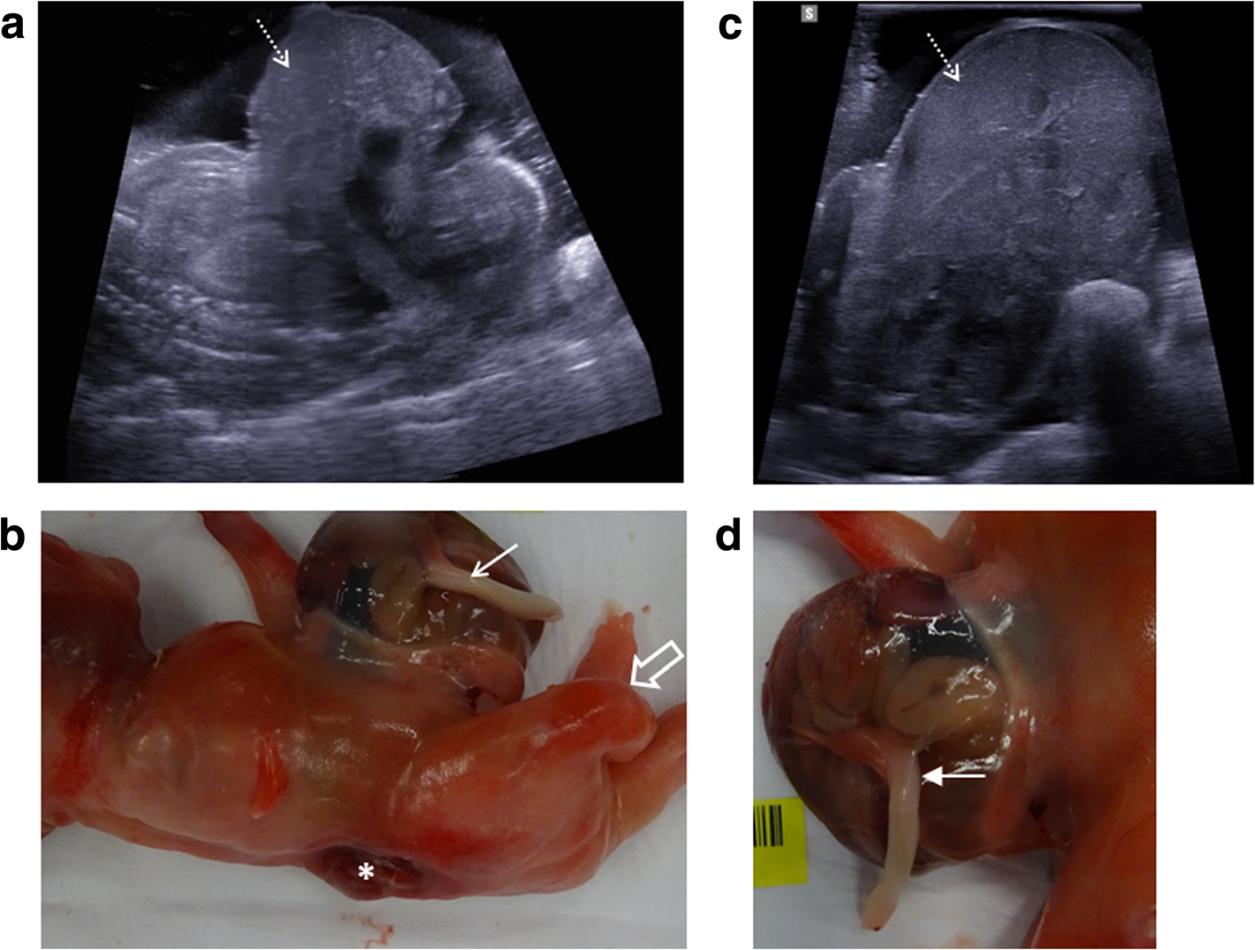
Large exomphalos in a foetus at 22 weeks gestation, following termination of pregnancy for multiple congenital anomalies. Post-mortem ultrasound performed 10 days after delivery in both sagittal (a) and transverse plane (c) demonstrate herniation of bowel contents through the anterior abdomen. The contents of the exomphalos contained loops of bowel and the majority of the liver (dotted arrow). The external photographs of the foetus at autopsy (b, d) show the herniated contents being covered by a thin membrane with the umbilical cord arising from this (solid arrow). It is also possible to identify further anomalies of a neural tube defect (asterisk) and hypoplastic right limb (unfilled arrow) from the external images
